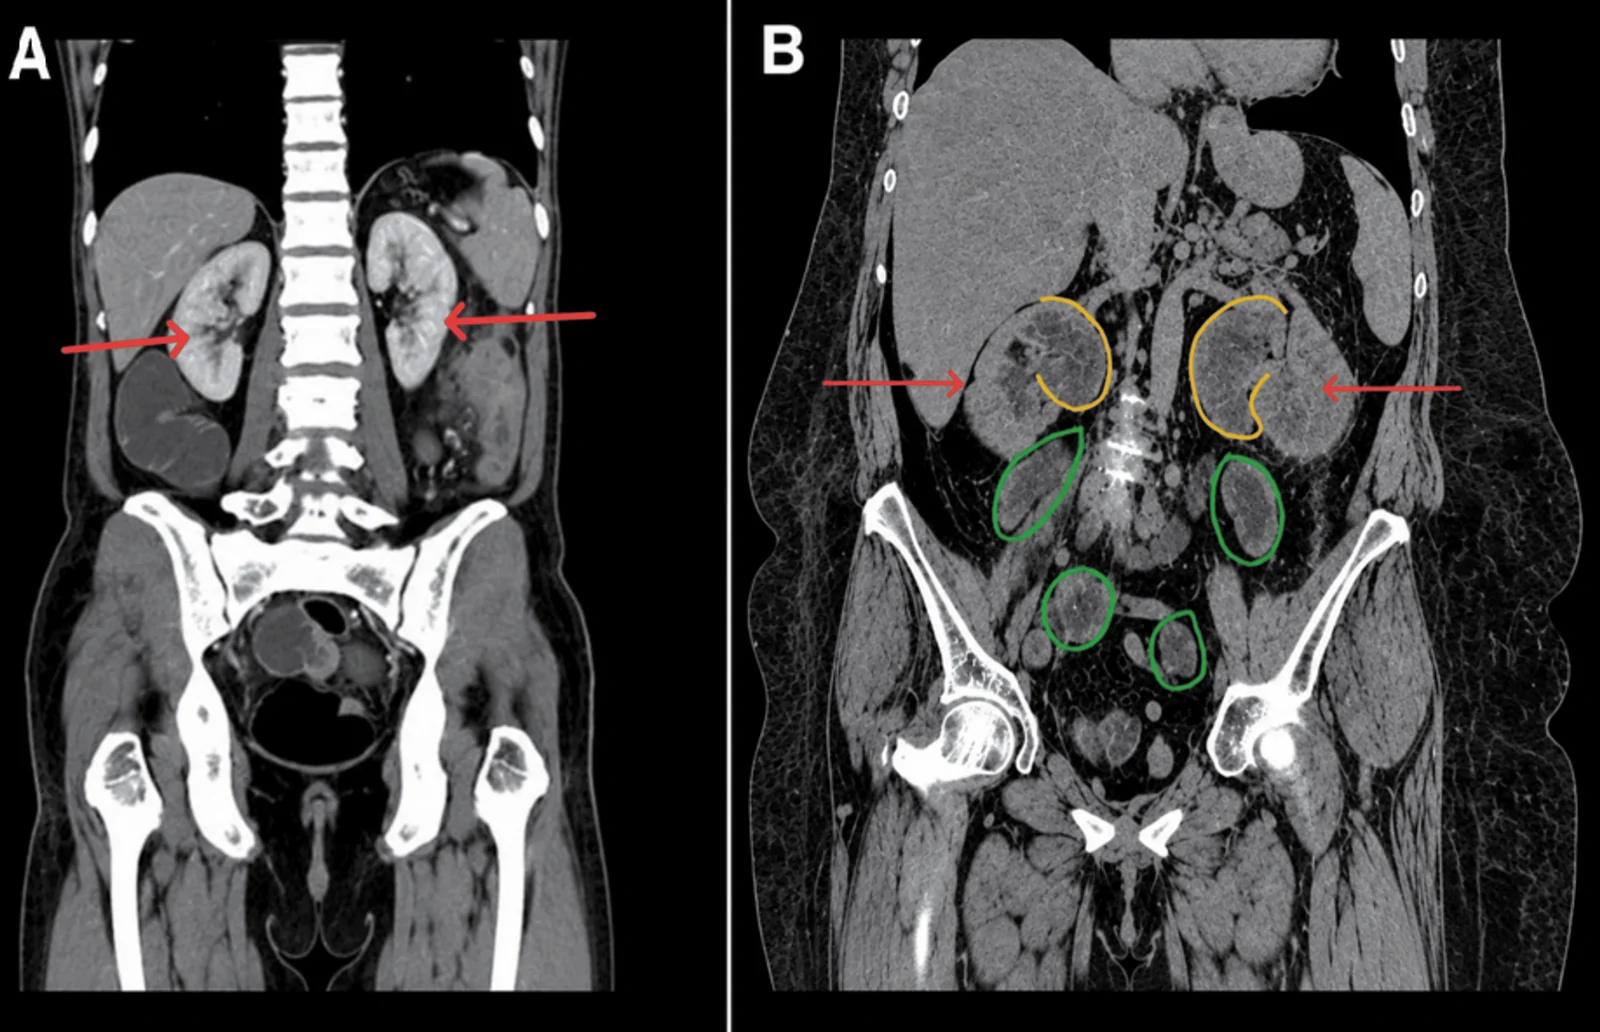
Coronal view of CT abdomen (A) Normal kidneys (red arrows) without hydronephrosis. (B) Bilateral moderate hydronephrosis (yellow line), bilateral hydroureter (green circles), and kidneys (red arrows) CT: computed tomography
